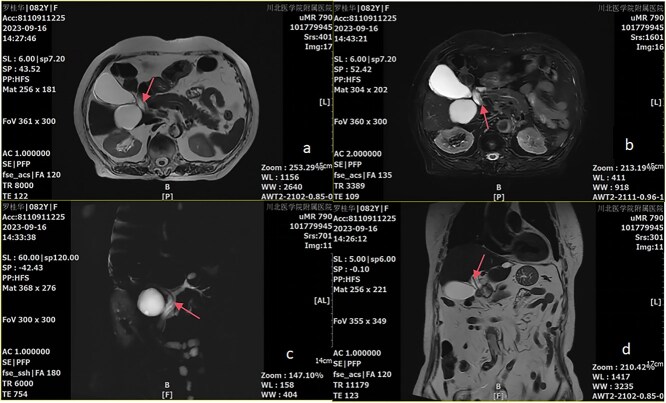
Magnetic resonance T2-weighted imaging (T2WI) (abcd)(red arrows point to the roundworm imaging finding in the common bile duct).
